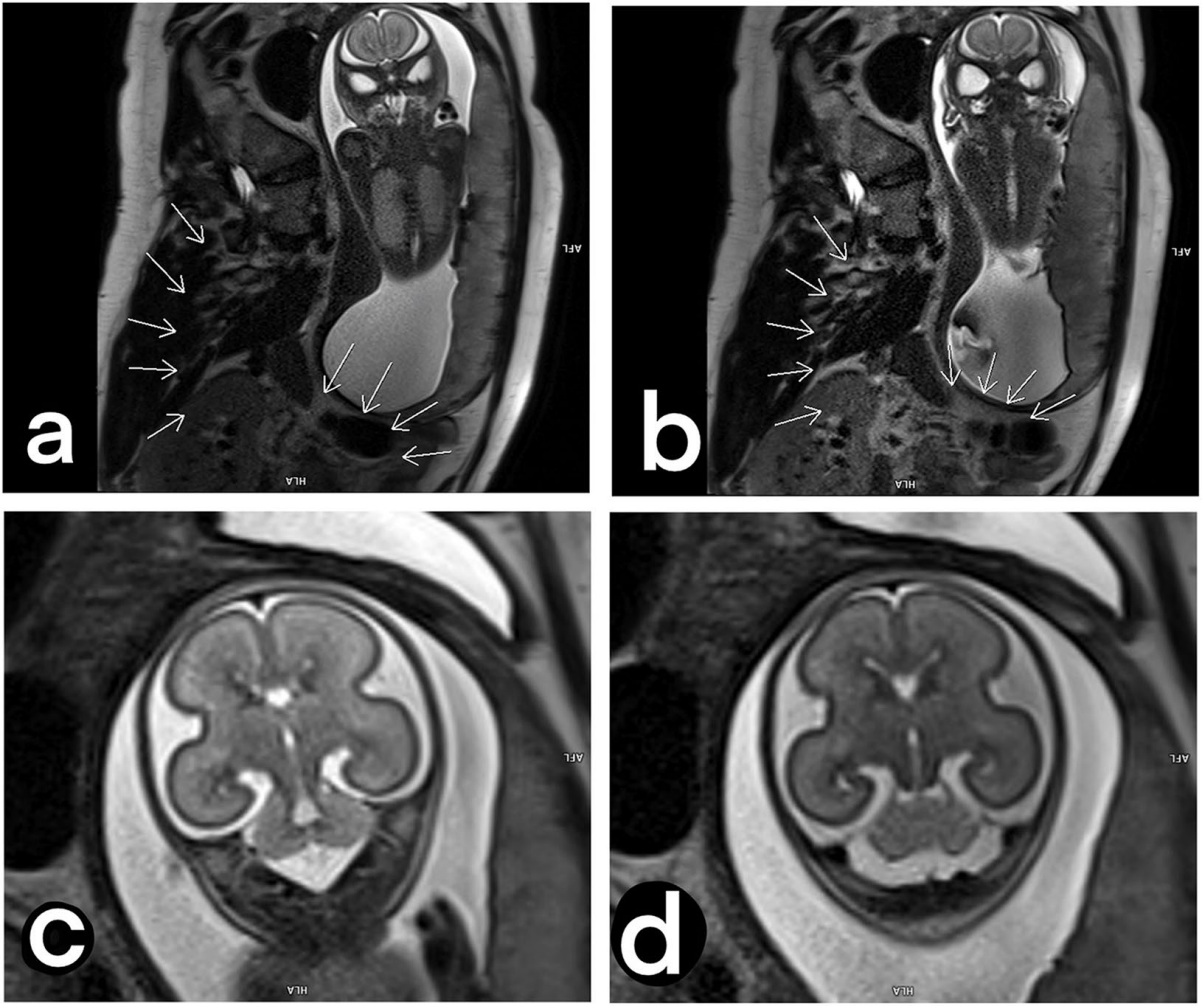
Coronal views of a fetal body before and after the setting of a prescan-B_1_ filter for T2-weighted single-shot fast spin-echo acquisitions, to describe dielectric artifacts. The signal intensity is spread to the image after the B1 filter was applied, showing up details around the mother’s organs (a, b). Images focused on the fetal brain, to describe how the body part of interest could be affected by the B_1_ filter: no B_1_-filter applied (c). B_1_-filter applied (d)
